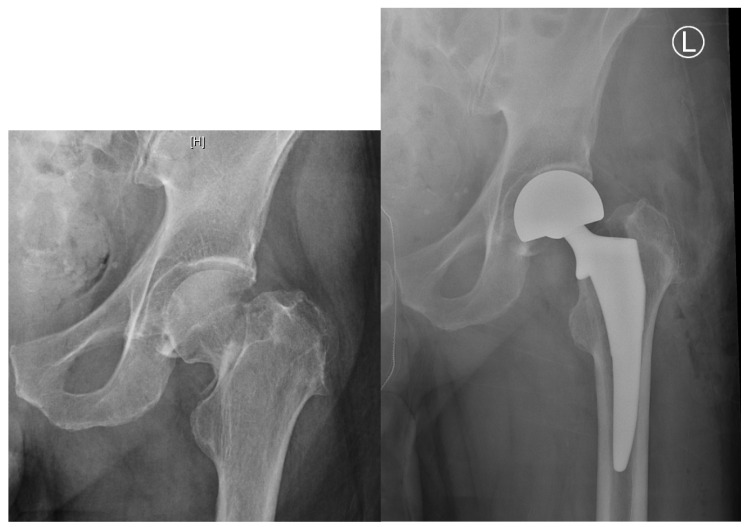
Example radiographs demonstrating total hip arthroplasty Garden III fracture (Left: Preoperative, right: Postoperative).
